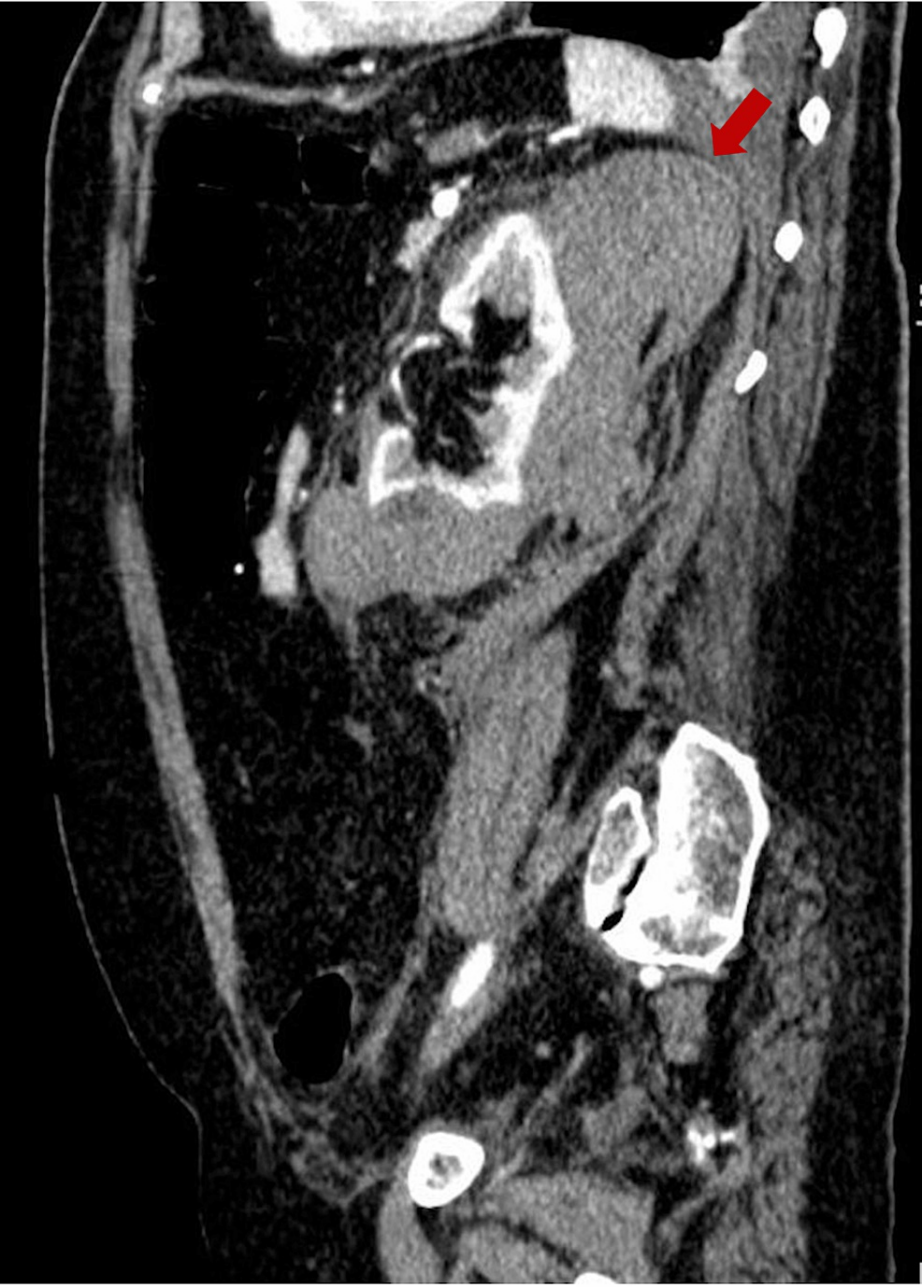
Abdominal computed tomography scan displayed the presence of a perirenal hematoma on the left side, as indicated by the red arrow (sagittal view).
